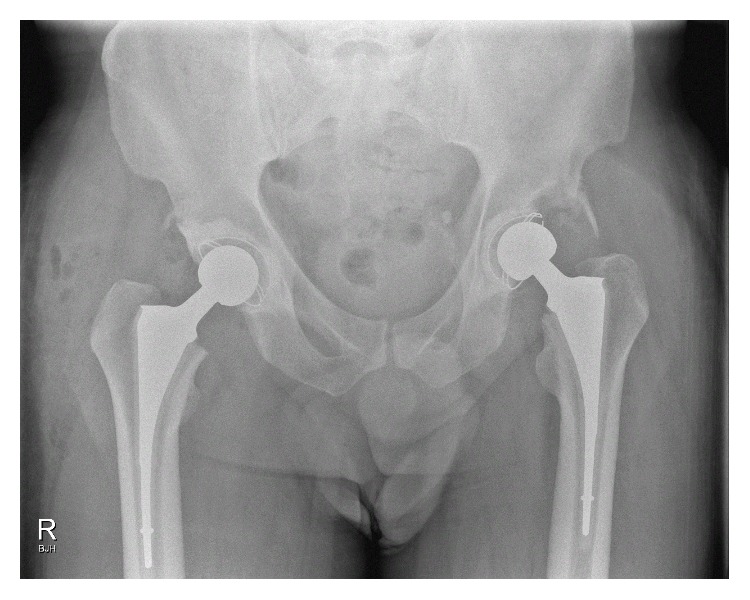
Postoperative radiograph demonstrating bilateral first-stage cemented total hip replacement.
